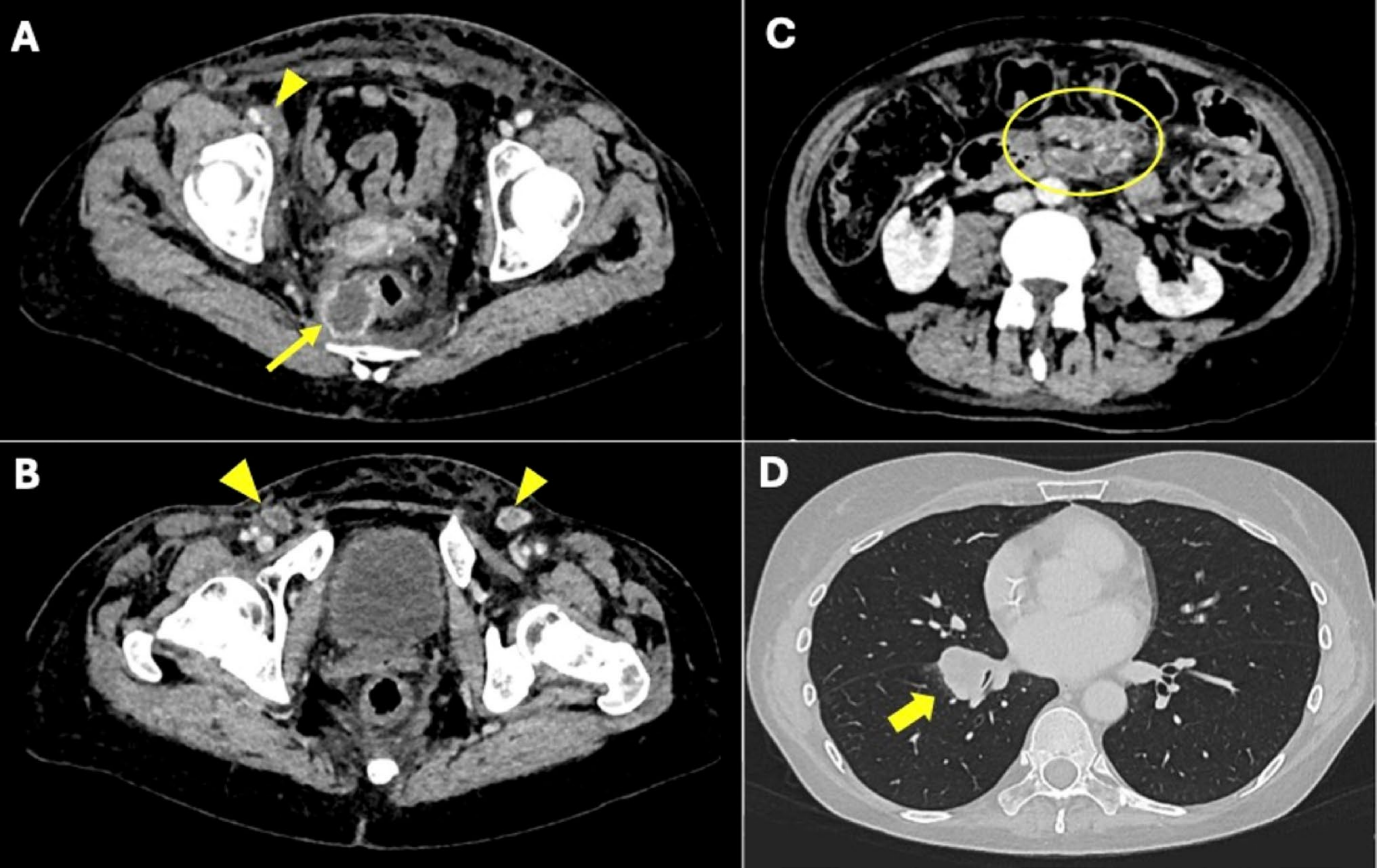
Representative CT findings of patient 1, of the abdomen and lung at her last progression after local radiation and ongoing Pembrolizumab. A-C: Axial, soft tissue window CT-scans and D axial lung window CTs. A: right perirectal hypodense tumor, suspicious of a perirectal lymph node metastasis (yellow arrow). B: inguinal lymph node metastases (yellow arrow heads). C: large lymph node metastasis of the mesentery (yellow circle). D: large pulmonal metastasis with compression of the right lower lobe bronchus (yellow arrow)
